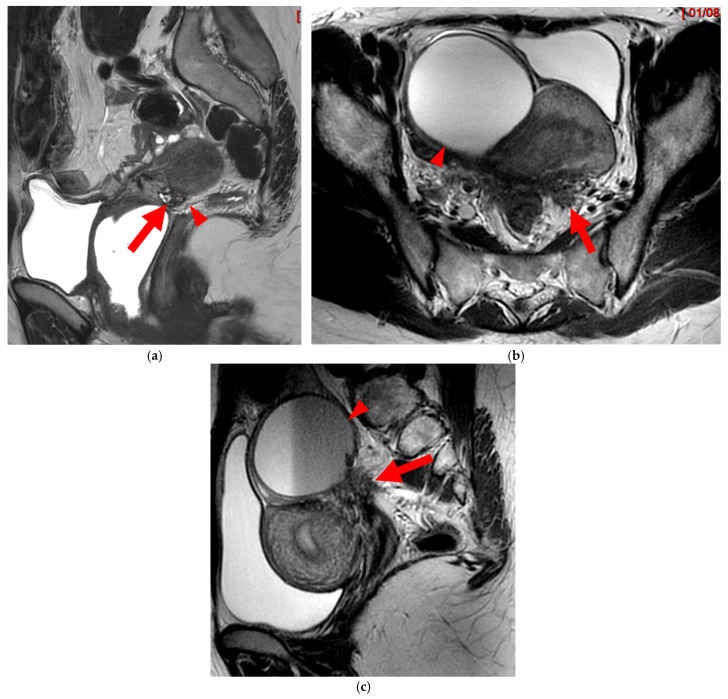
Pelvic MRI scans of two patients with HTD type 5B USLs. (a) Patient 1: sagittal T2WI shows a microcystic nodule (*arrow*) within the origin of the right USL (*arrowhead*). (b,c) Patient 2: axial (b) and sagittal (c) T2WI show a nodular left USL with spiculated margins (*arrow*) and a right ovarian endometrioma (*arrowhead*).
